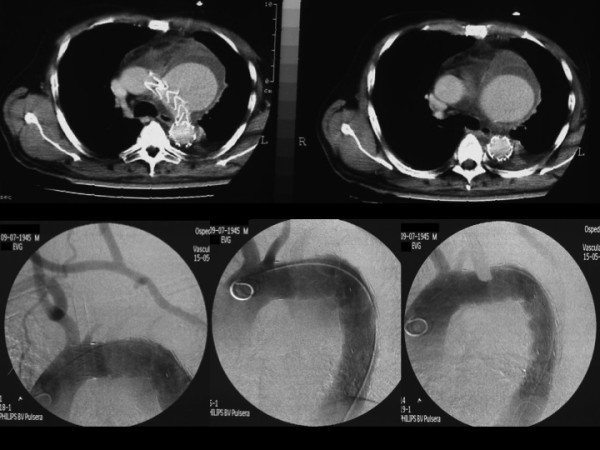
CT and angio images of displacement of the graft and enlargement of the sac.
